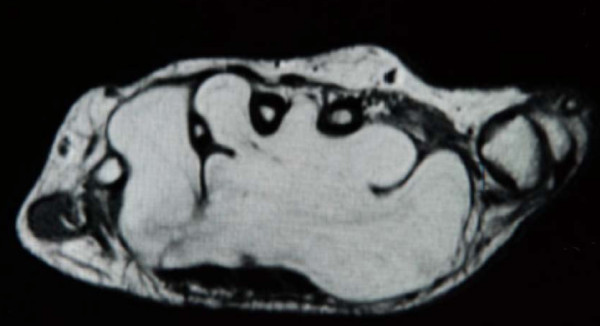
MRI showing the recesses of the lipoma, invading the web spaces between the metacarpals.
